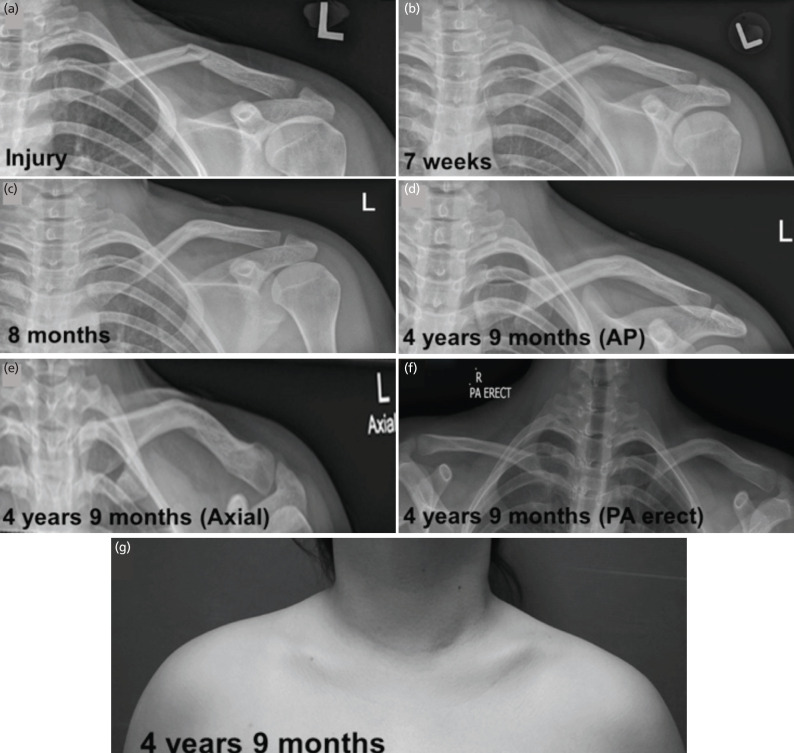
Radiographs and clinical photo of a 15-year-old female with a displaced midshaft left clavicle fracture. (a) First radiograph of injury, (b) AP radiograph at seven weeks, (c) AP radiograph at eight months, (d) AP radiograph at four years nine months, (e) Axial radiograph at four years nine months, (f) EOS radiograph at four years nine months, allowing comparison with non-fractured right clavicle, (g) clinical photo at four years nine months.
